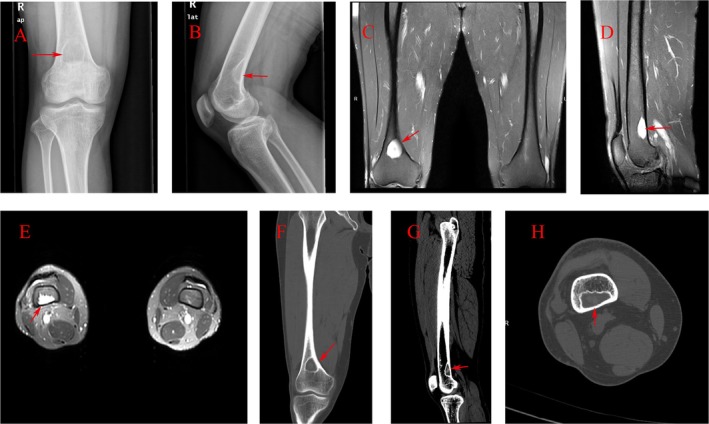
Imaging findings of the initial liposclerosing myxofibrous tumor (LSMFT) in the right distal femur. (A,B) Anteroposterior and lateral radiographs showing a benign‐appearing cystic lesion in the distal femoral metaphysis (red arrows). (C–E) Magnetic resonance images (C, coronal T2‐weighted; D, sagittal T2‐weighted; E, axial T2‐weighted) showing a cystic lesion at the distal femur (red arrows). (F–H) Computed tomography images (F, coronal; G, sagittal; H, axial) showing a 1.4 cm × 2.8 cm × 3.2 cm benign‐appearing lesion of suspected fibrous origin (red arrows).
